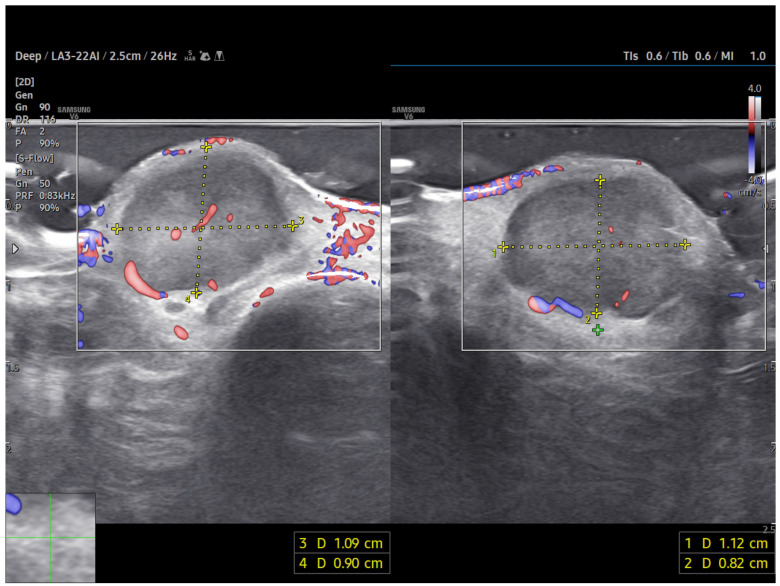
US imaging of MCC. The lesion appeared as a well-circumscribed, homogeneous, subdermal mass resting on the extensor tendon of the third finger on the dorsal ulnar side. On ultrasonography, the mass showed a finely heterogeneous echotexture with vascularization on power Doppler, both peripheral and central. One vascular pole appeared to supply the lesion via a central vessel.
